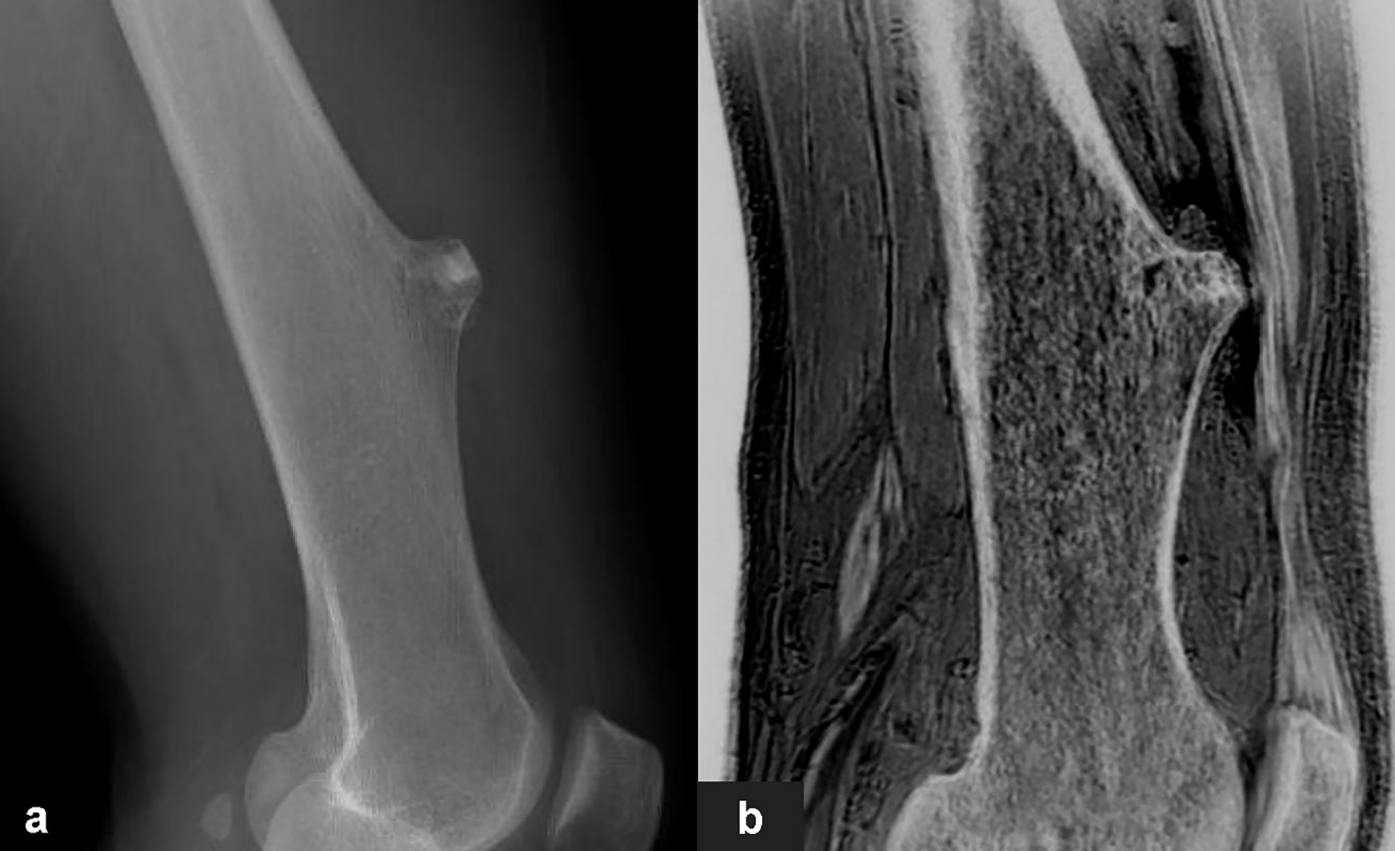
Images in a 65-year-old male with osteochondroma of the left lower femoral shaft. (A, B) were lateral X-Ray and sagittal FRACTURE images. Wide base and morphology of tumor were consistent in X-Ray and FRACTURE images.
